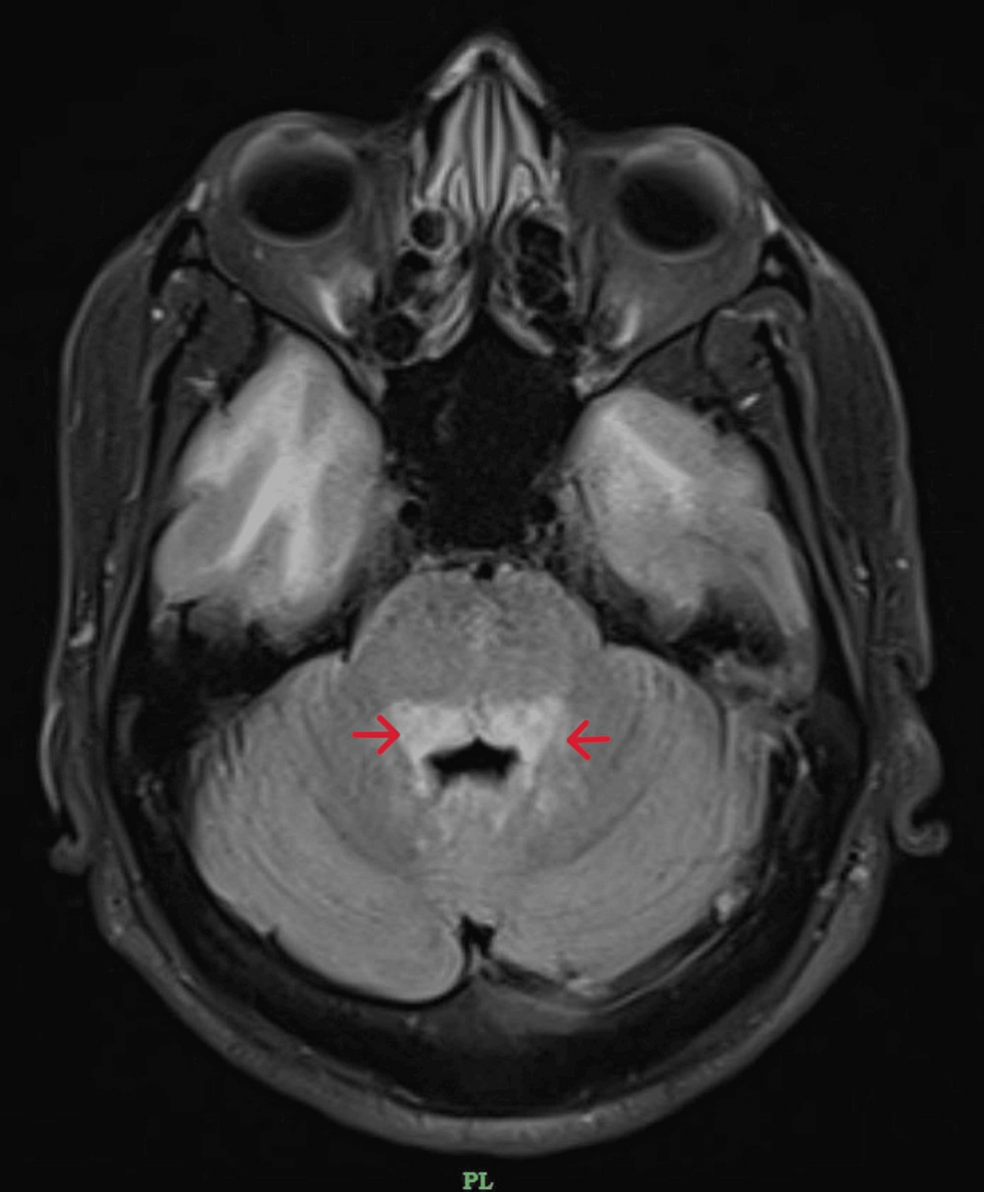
MRI brain fluid-attenuated inversion recovery (FLAIR) - Diffuse areas of altered signal intensities in dorsal midbrain pons superior and inferior cerebellar peduncles
